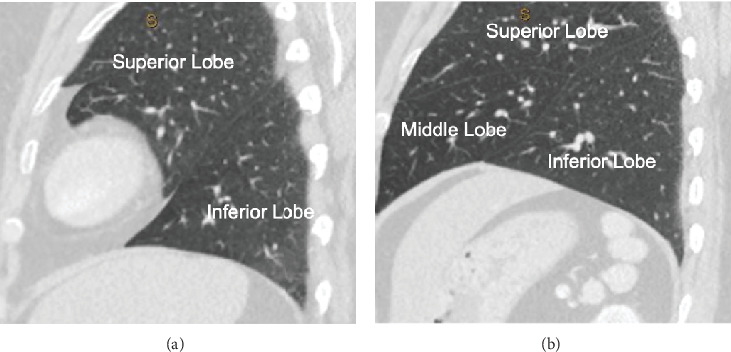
Sagittal CT shows (a) the normal configuration of the bilobed left lung and (b) the normal configuration of the trilobed right lung.
